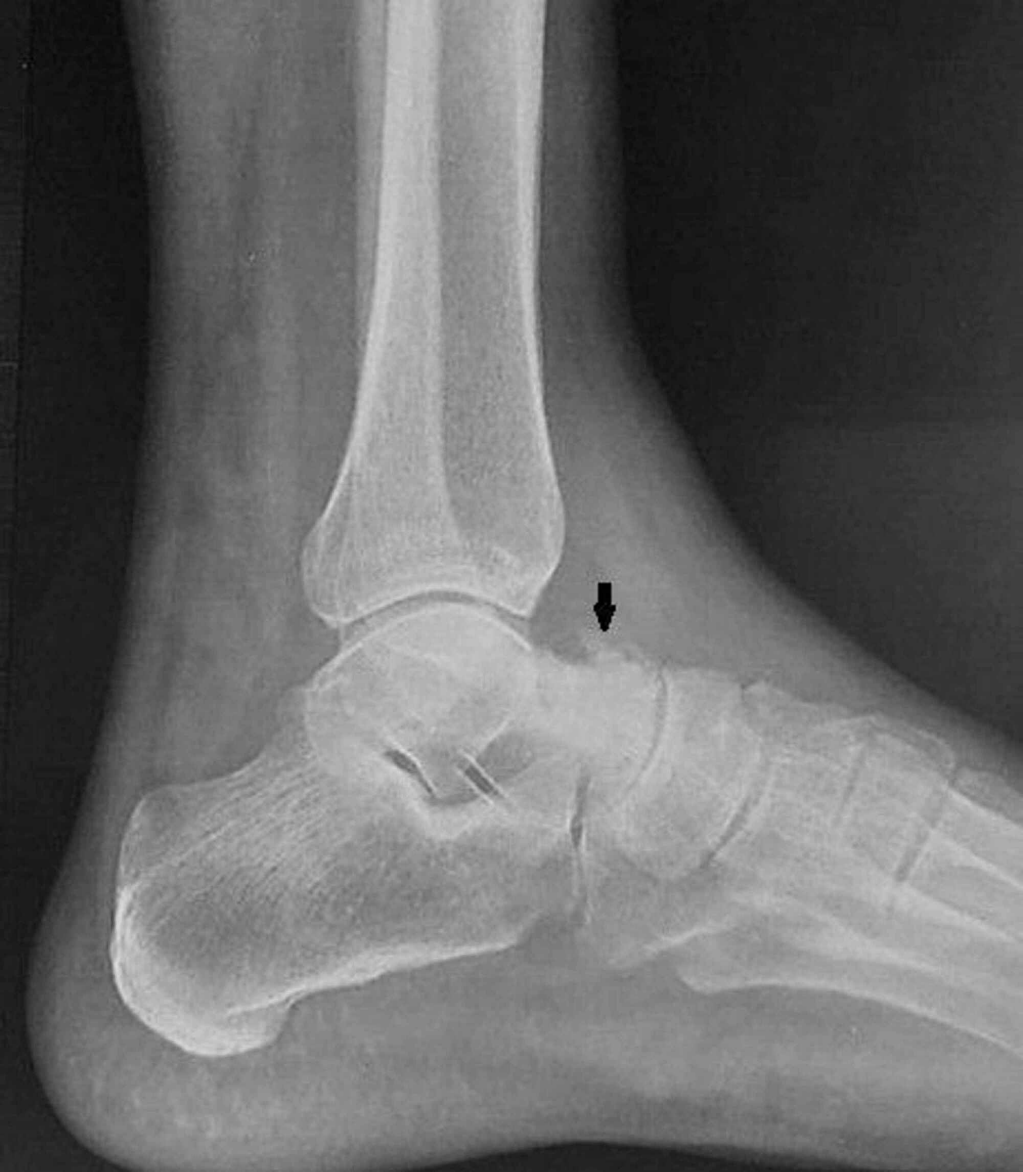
Lateral radiograph of the ankle and hindfoot demonstrates an exophytic sclerotic osseous lesion at neck of talus with punctate calcifications (black arrow)
